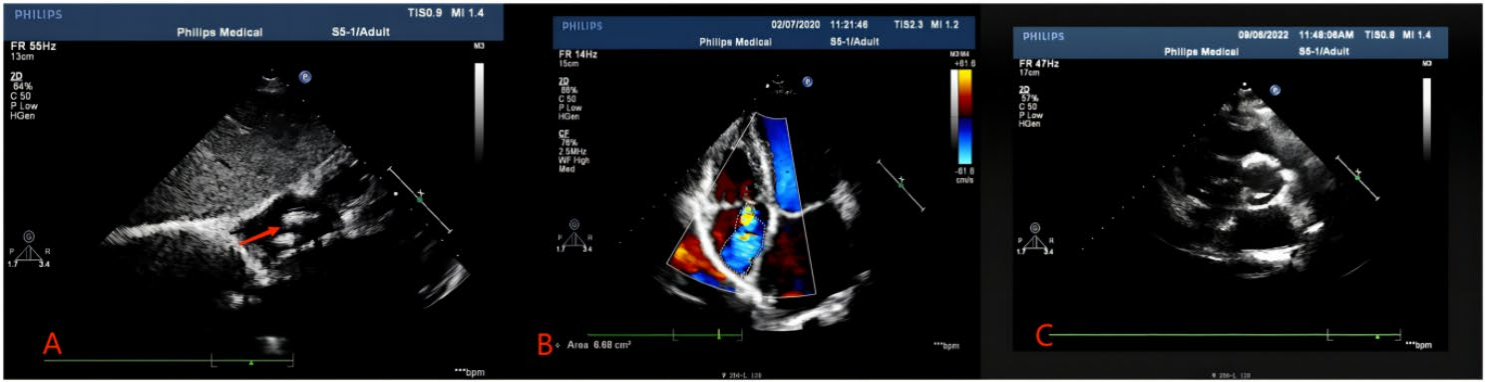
Transthoracic echocardiography images of hemangioma, (A) Two hyperechoic masses in the right atrium (red arrow), (B) Preoperative echocardiography, (C) Postoperative echocardiography
